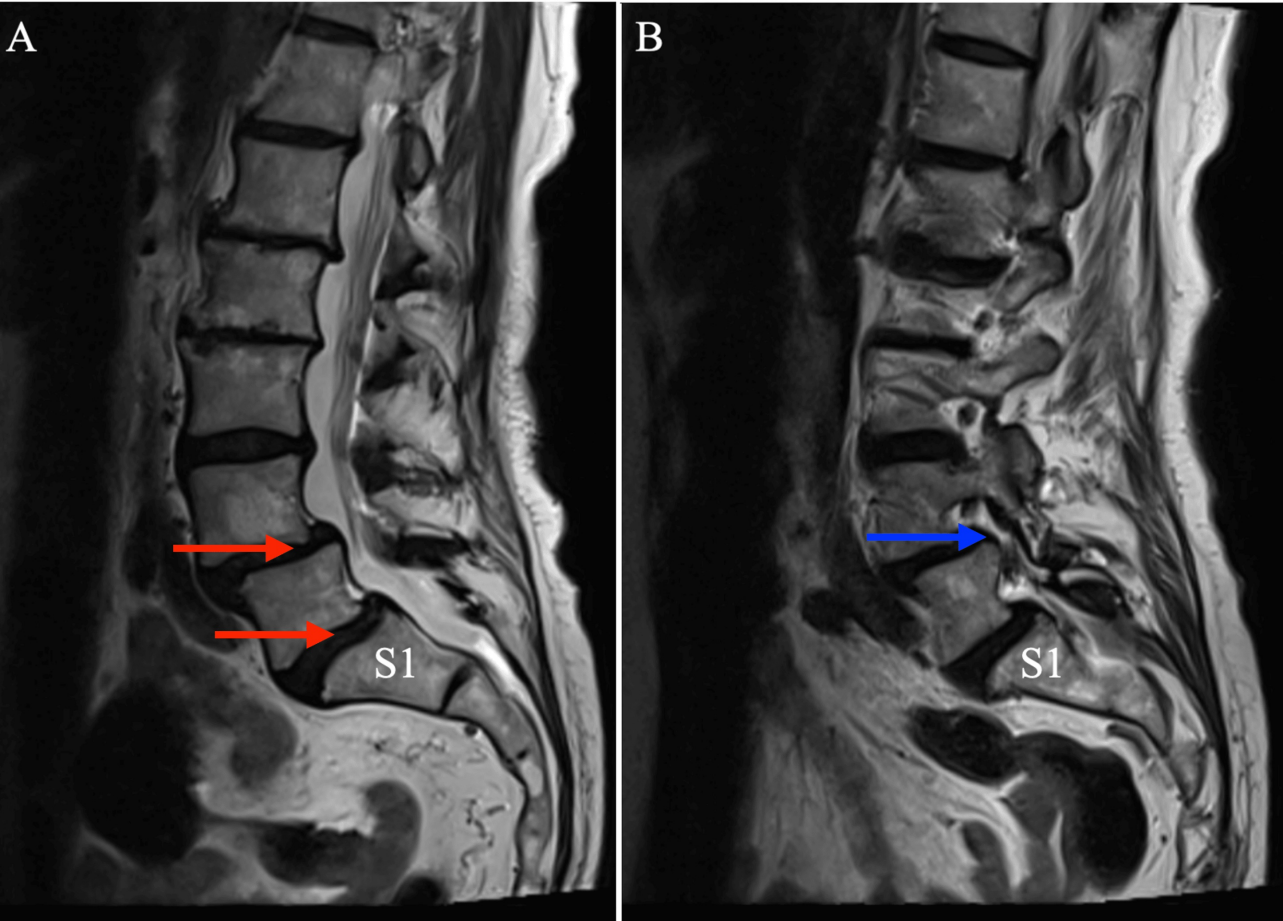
Pre-operative lumbar T2 sagittal MRI demonstrating significant degenerative spondylosis of L4-S1 levels (A), and severe lateral stenosis at L4-L5 (B). The S1 vertebrae is labeled "S1." The L4-S1 degenerative change is highlighted with red arrows and the lateral stenosis is delineated with a blue arrow.
